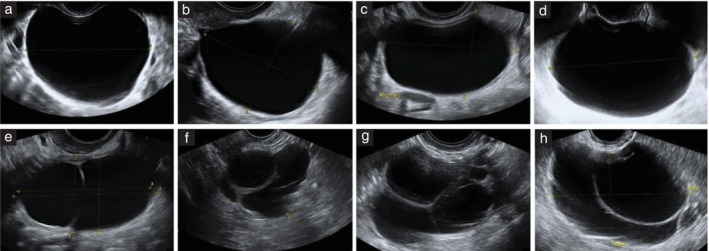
Grayscale ultrasound images showing typical features of serous cystadenomas. (a–d) Unilocular anechoic cysts with smooth walls. (e–h) Multilocular cysts showing thin septations and < 10 cyst locules.
